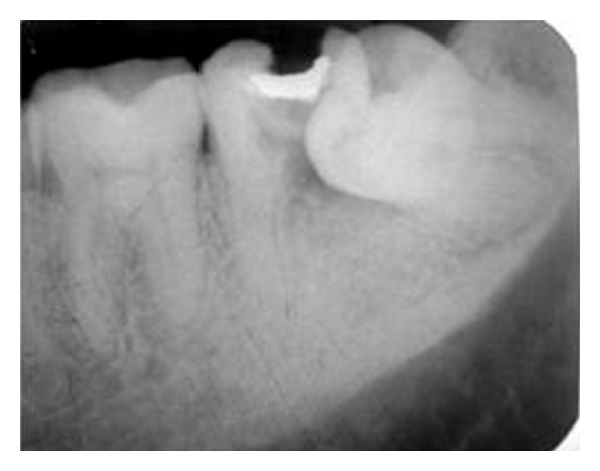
Preoperative radiograph showing 36, 37 and 38.
